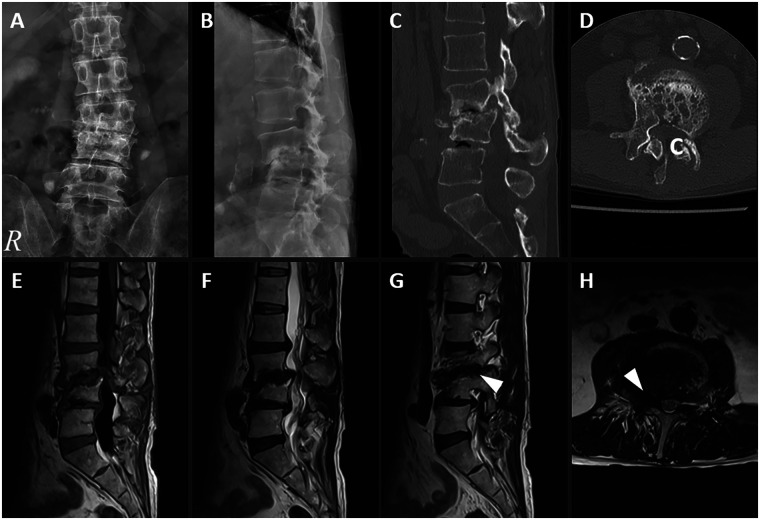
Preoperative imaging studies of the lumbar spine. Plain radiography (A,B) and computed tomography imaging (C,D) of the lumbar spine showed destructive erosion of endplates, lytic lesions over vertebral bodies and spondylolisthesis at the L3−4 spinal segment. Magnetic resonance imaging showed anterior slippage of the L3 vertebrae over L4 (E,F), and posterior herniation of disc with severe stenosis (arrowhead) of the right L3−4 intervertebral foramen (G,H).
